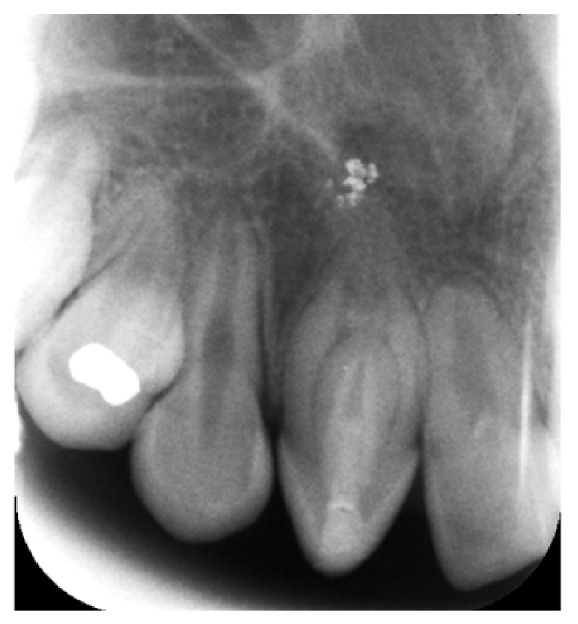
The one-year follow-up radiograph showing complete healing of the periapical lesion.
